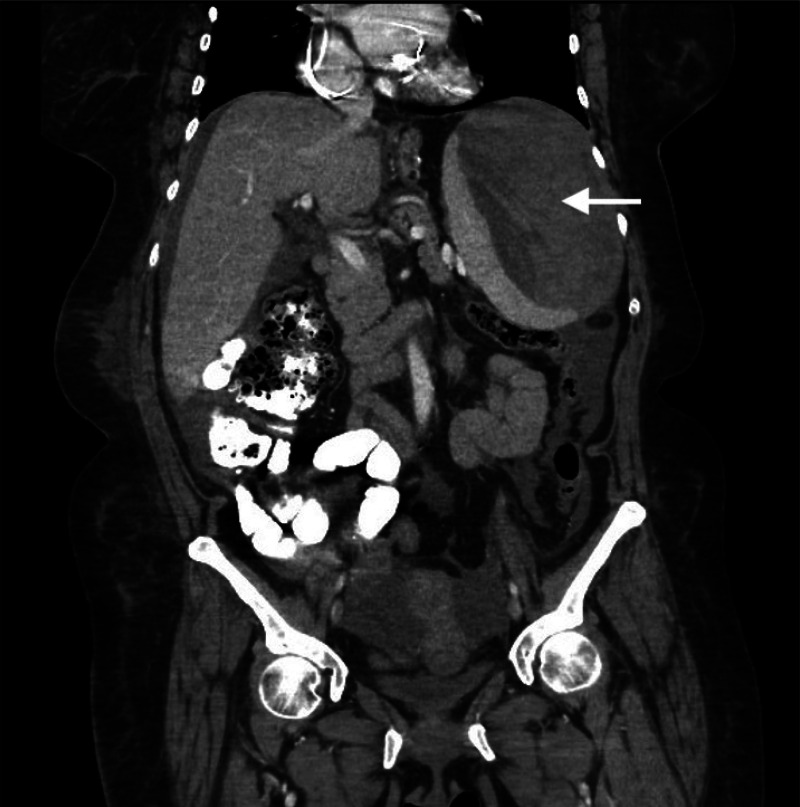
Contrast-enhanced coronal computed tomography of the abdomen demonstrating a large (15 × 9 × 15 centimeter) perisplenic hematoma (arrow) with concomitant hemoperitoneum due to atraumatic rupture.
